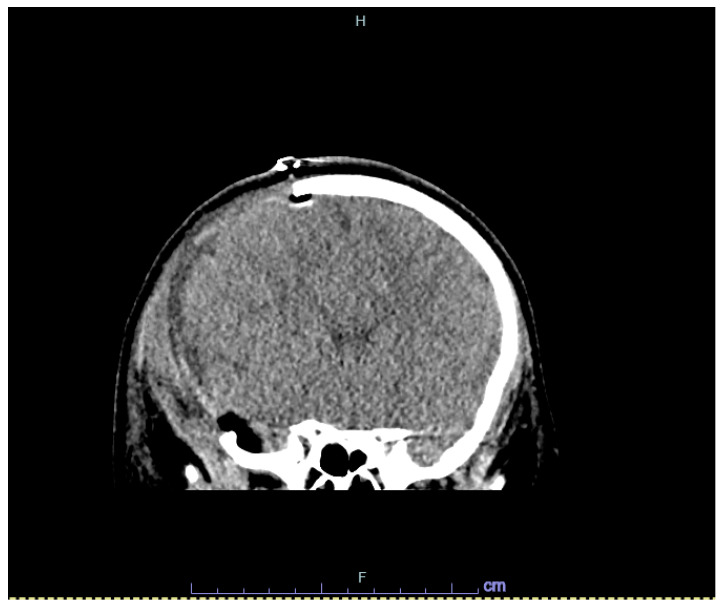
Post-hemicraniectomy head CT. Coronal view after subdural hematoma evacuation.
